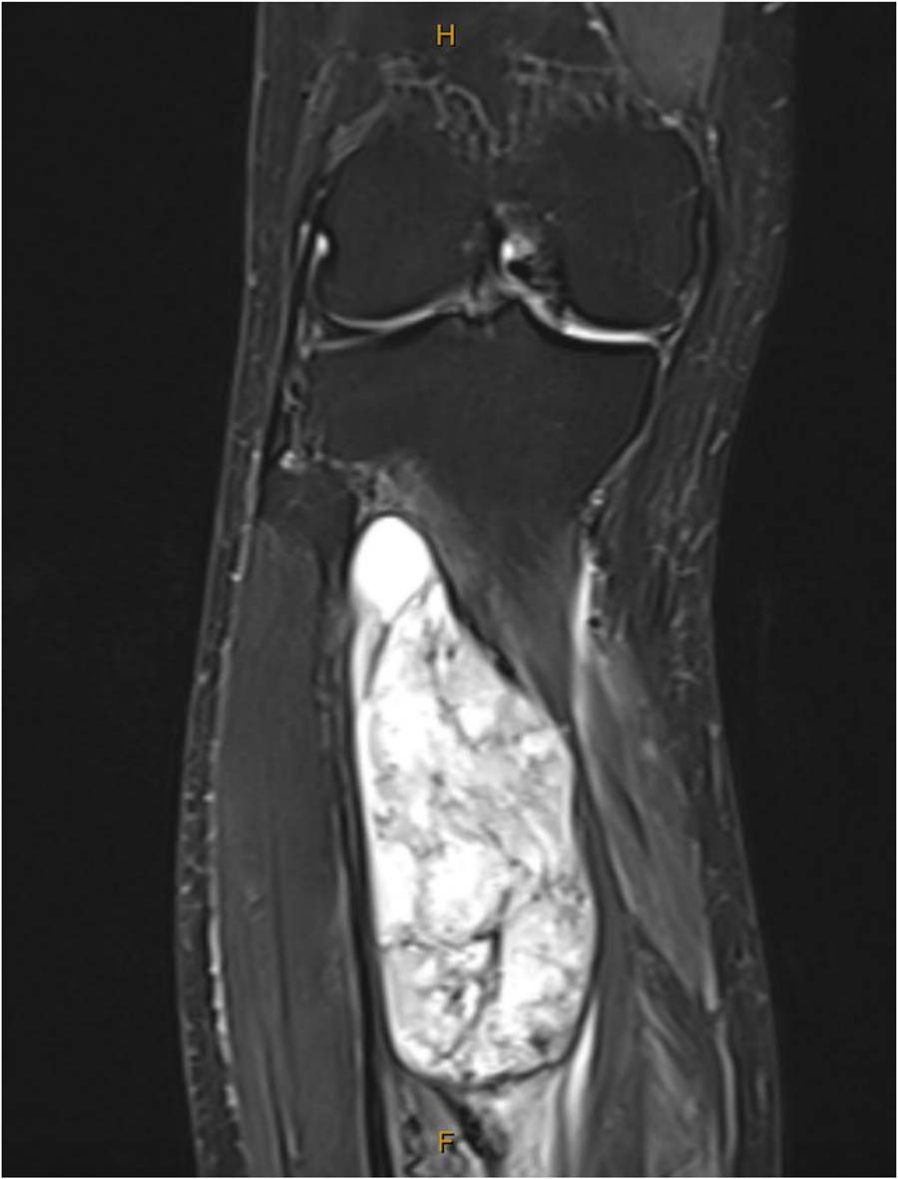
MRI showed a large tibialis posterior intramuscular lesion with proximal tibial involvement.
